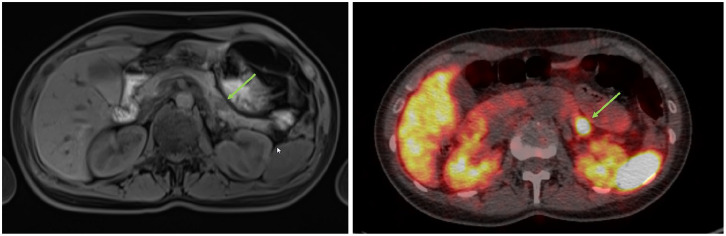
Imaging of our patient, female, 35-years. Left side: MRI scan T1 vibe native, axial. Right side: GA-Dotatoc-PET 3.3 mm, axial. Both imaging methods were performed at an external clinic and provided from the patient who gave her written consent for publishing. The green arrow marks the lesion of the pancreatic tail.
